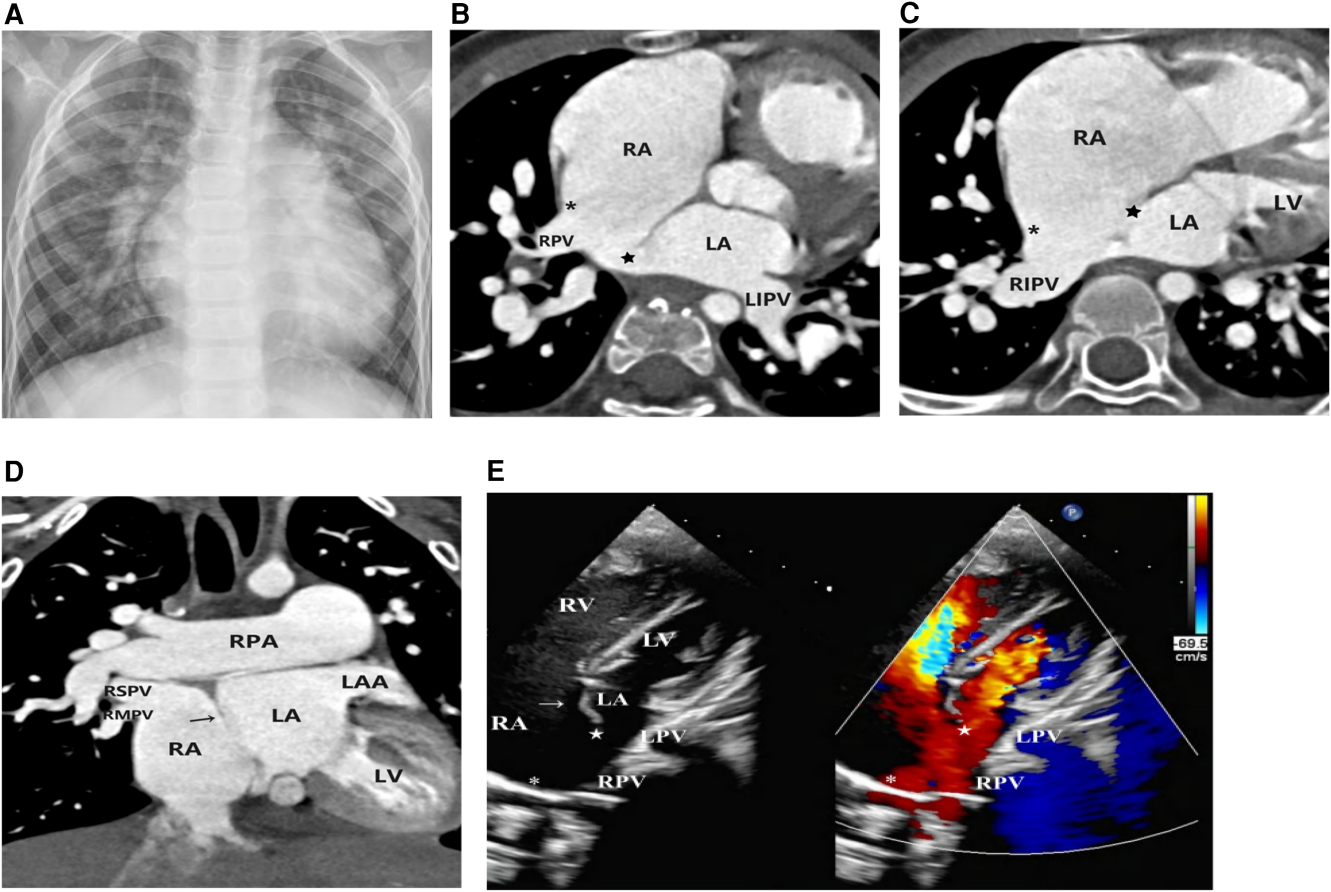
Imaging results for Case 2. (A) Chest x-ray: The heart was enlarged with a rounded and blunt apex. The pulmonary vascular markings were increased and coarse, accompanied by flocculent shadows. (B–D) Axial and coronal view of CTA: The right atrium was enlarged. The left superior and inferior pulmonary veins drained into the left atrium, while the right superior and inferior pulmonary veins drained into the anatomical right atrium (accessory atrium). A localized defect (
